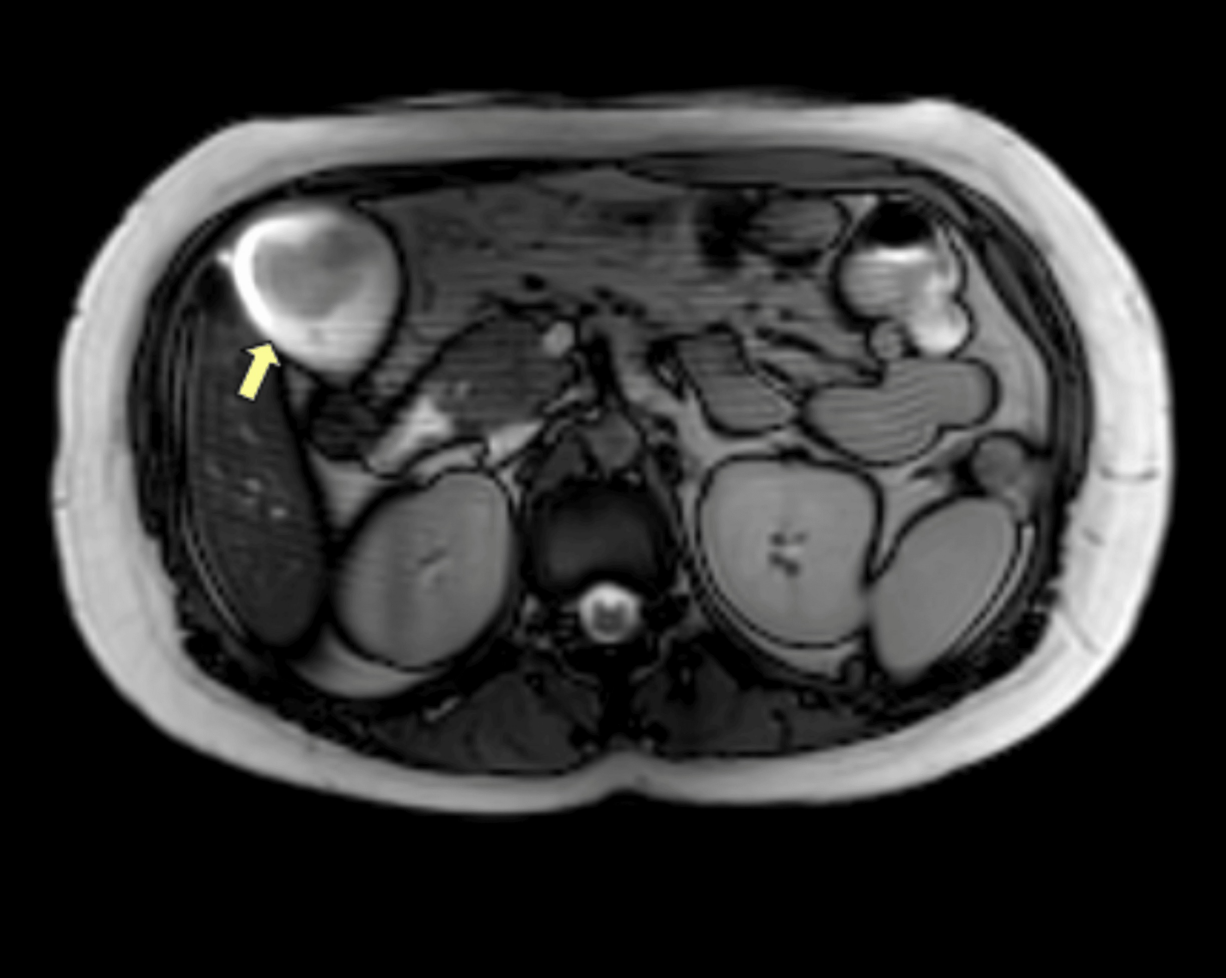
Contrast-enhanced magnetic resonance cholangiopancreatography demonstrating a fundal gallbladder lesion. Magnetic resonance cholangiopancreatography in axial view showing the gallbladder fundal mass with intermediate signal intensity on T1- and T2-weighted sequences, adherent to the gallbladder wall without extension into the pericholecystic planes (yellow arrow).
